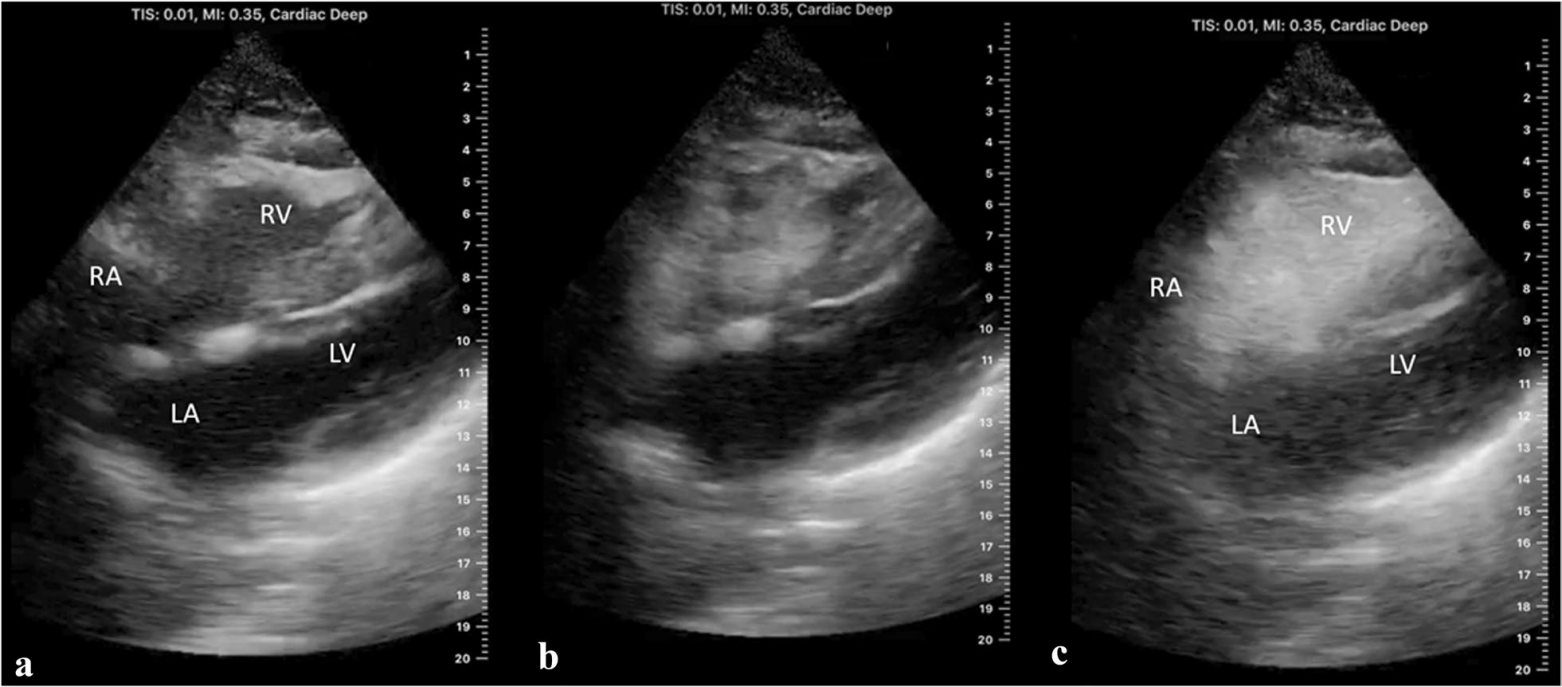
Intrapulmonary shunt in a mechanically ventilated patient with consolidated lung. Intrapulmonary shunt in a mechanically ventilated patient with consolidated lung. a Labeled 2-D subcostal 4-chamber transthoracic echocardiographic (TTE) view of the heart. *RA* right atrium, *RV* right ventricle, *LA* left atrium, *LV* left ventricle. b 2-D subcostal 4-chamber echocardiographic view of the heart after i.v. injection of saline microbubble contrast showing the arrival of the contrast in the RA and RV. c Labeled 2-D subcostal 4-chamber echocardiographic view of the heart after i.v. injection of saline microbubble contrast demonstrating grade 3 shunt (> 25 bubbles). Bubbles appeared in the left ventricle 5 cardiac cycles after opacification of the right ventricle suggesting the presence of an intrapulmonary shunt
